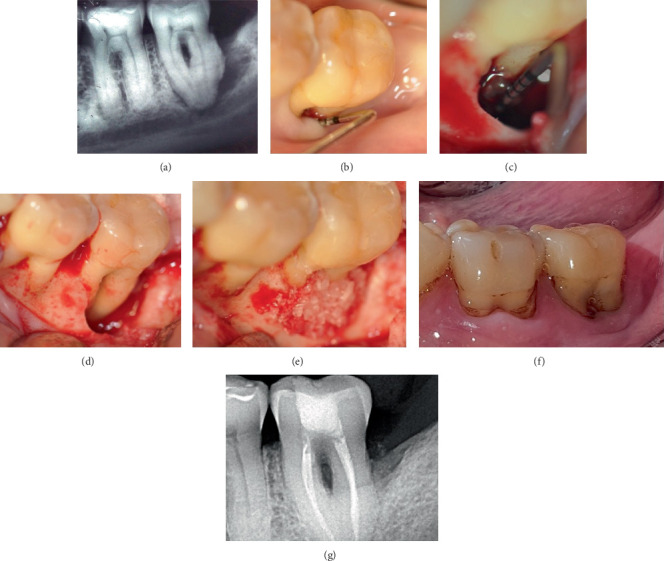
Clinical presentation of Case 4. (a) Preoperative periapical radiograph showing severe bone loss extending to the apex of Tooth #37, with a Class III furcation lesion and a short root trunk. (b) Initial probing depth, demonstrating a 14-mm periodontal pocket. (c) Approach was achieved by raising a mucoperiosteal flap, calculus was present on the root surfaces, and a large loss of periodontal support was evident. A 12-mm intrabony periodontal defect was observed. (d) Surgical root surface debridement was performed. Class III furcation involvement of the mandibular left second molar. (e) Intrabony defect was treated with a combination of enamel matrix derivative (EMD) and allograft materials. (f) Clinical view 6-year follow-up. (g) Although the furcation lesion persists, an improvement in the initial distal bone defect area can be observed.
